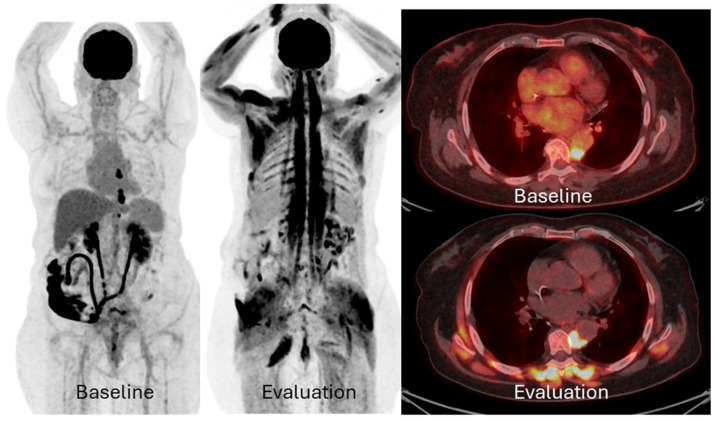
MIP and PET/CT fused images of a patient at baseline and follow-up. She had thoracic peri-aortic metastatic lymphadenopathy that increased in intensity and size between baseline (maximum standard uptake value (SUVmax) 9.7; 18 mm) and evaluation FDG-PET/CT after 3 cycles of Pembrolizumab (SUVmax 14.6; 21 mm). Note that lesion’s FDG uptake on MIP images of evaluation FDG-PET/CT is masked by intense muscular uptake due to immune-related adverse effect of Pembrolizumab that led to adrenal insufficiency. She was classified as progression with PERCIST and imPERCIST5, and as stable disease with PERCIMT. She did not progress until 16 months of Pembrolizumab treatment, and we concluded that she had pseudoprogression. Treatment for adrenal insufficiency was initiated.
